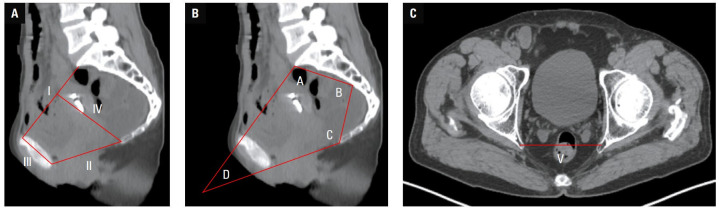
Computed tomography (CT)-­based pelvimetric parameters; A, B – pelvic sagittal CT images; pelvic inlet anteroposterior diameter (I); pelvic outlet anteroposterior diameter (II); pubic symphysis height (III); pelvic depth (IV); letters A–D represent pelvic angles. C – pelvic cross‑sectional CT image; bispinous diameter (V)
